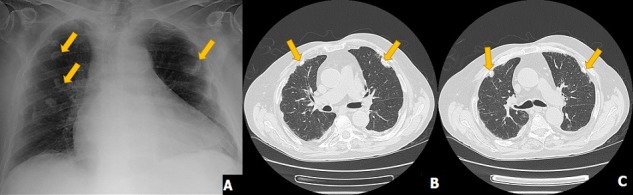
(A) chest radiograph (CRX) revealed showed cardiomegaly, signs of bibasal congestion and well delimited focal areas suggestive of pleural calcifications; (B, C) high-resolution chest computerized tomography (HRCT) revealed multiple gross calcifications in axial sections with windows to the mediastinum, some of them associated with pleural thickening areas suggestive of pleural plaques (PP), without pulmonary parenchyma changes
